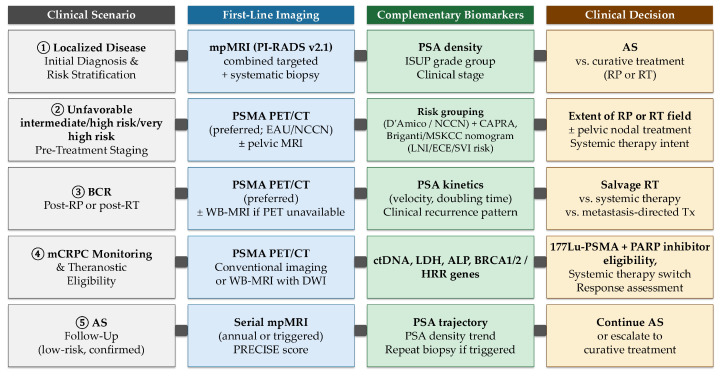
Clinical decision framework for biomarker and imaging tool selection across prostate cancer disease stages. Each lane maps a clinical scenario to the recommended first-line imaging modality, complementary biomarkers, and resulting clinical action. Lane 1 (initial diagnosis): mpMRI/PI-RADS v2.1 with combined targeted + systematic biopsy. Lane 2 (high-risk staging): PSMA PET/CT preferred per EAU/NCCN. Lane 3 (BCR): PSMA PET/CT first-line, WB-MRI when unavailable. Lane 4 (mCRPC and theranostic eligibility): PSMA PET/CT for ^177^Lu-PSMA-617 selection, with conventional imaging or WB-MRI when appropriate; HRR alterations for PARP eligibility. Lane 5 (active surveillance): serial mpMRI with PSA monitoring. Where PSMA PET/CT is unavailable, CT and ^99m^Tc bone scintigraphy remain the fallback. Supporting references for each lane are provided in the corresponding sections of the manuscript; abbreviations are defined in the manuscript abbreviations list.
